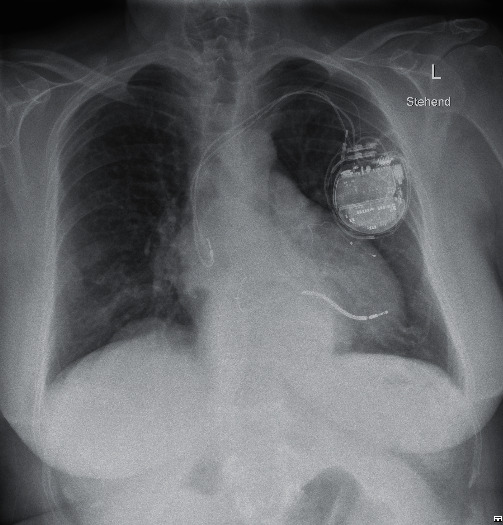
Post-implantation chest X-ray in posterior–anterior view.
